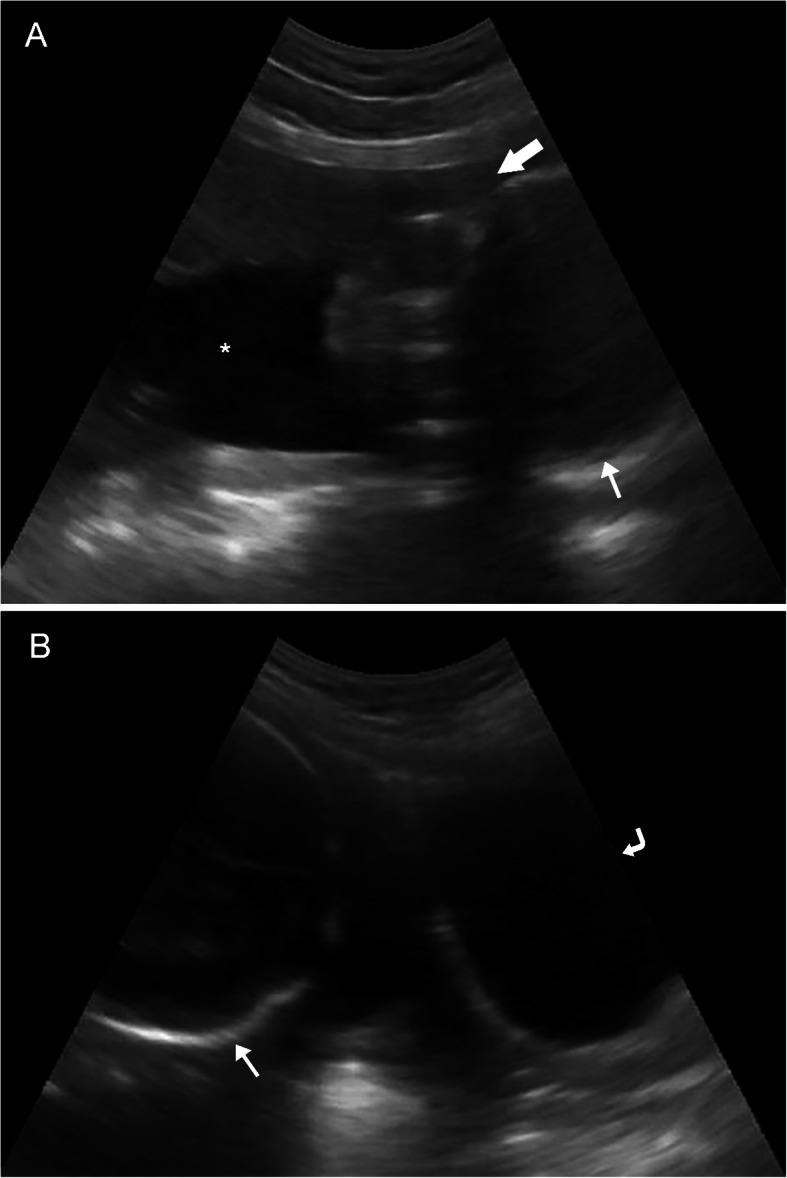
Example Images Acquired by Individuals Without Prior Training. a, b Still images from an ultrasound cine clip of a sagittal VSI sweep across the lower uterine corpus obtained by an operator without prior ultrasound training demonstrating a singleton early third trimester fetus in vertex presentation (thin arrow) with an anterior-mid placenta (block arrow) and grossly normal amniotic fluid volume (*). Maternal bladder is also seen in b (curved arrow)
